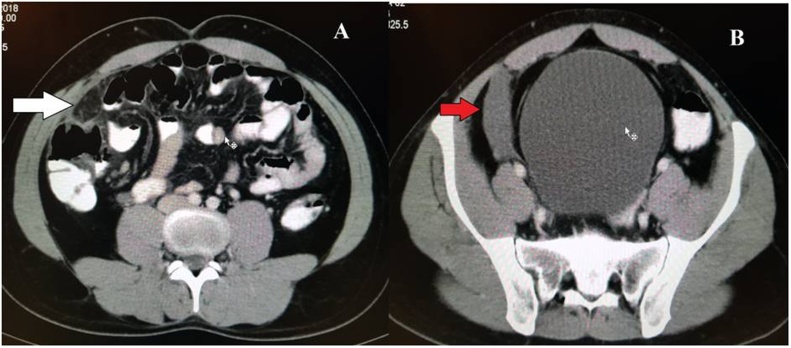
Contrast enhanced computed tomography showing omental fat stranding (white arrow) and free fluid in the right iliac fossa (red arrow).
